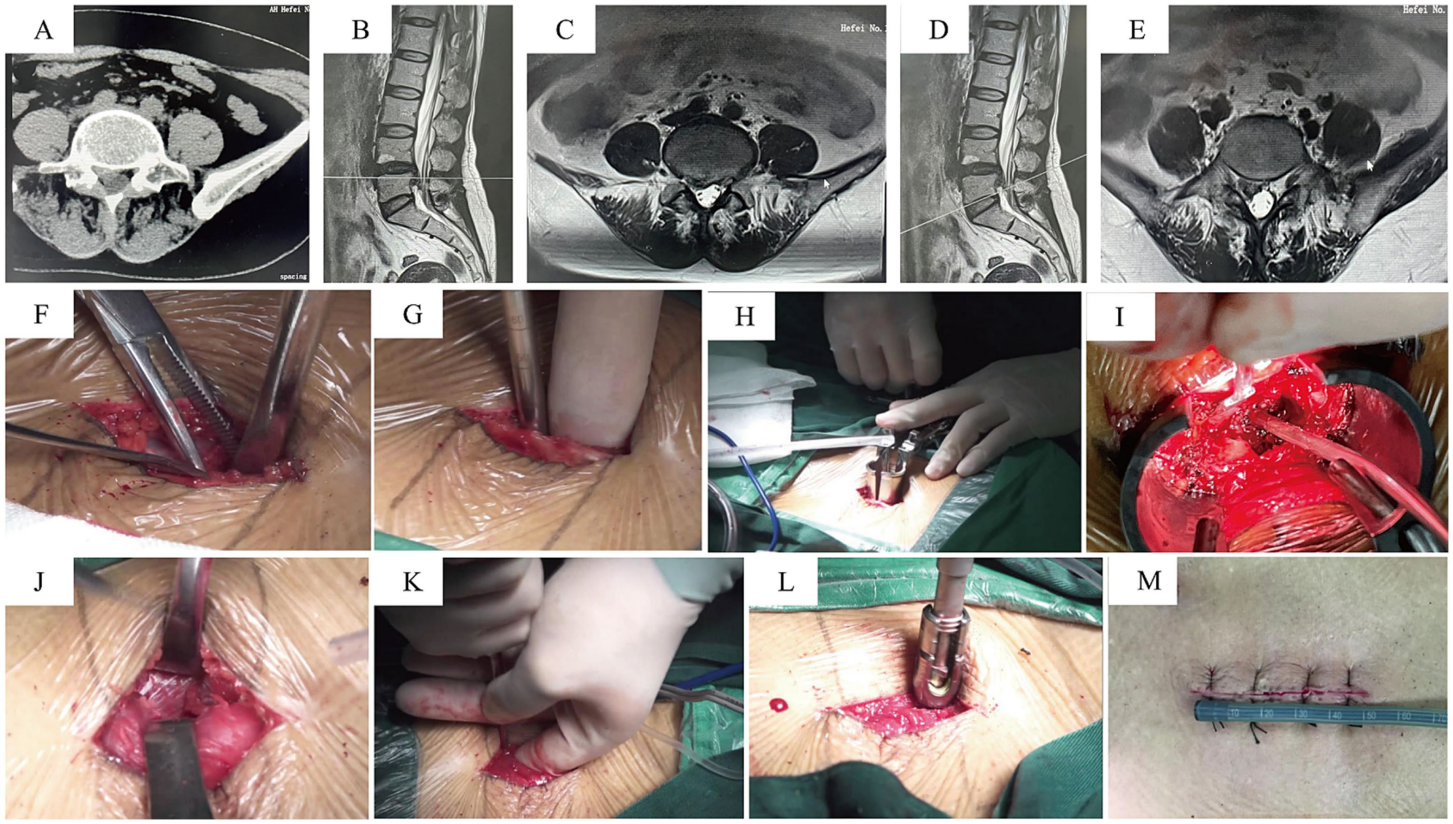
Representative Case 2. A 58-year-old female with low back pain and right lower limb numbness/pain for 2 years, aggravated for 2 months. (A–E) Preoperative CT and MRI: right-sided L4/5 lumbar disc herniation, migrated inferiorly, compressing the nerve root and causing foraminal stenosis. (F–H) A midline incision was used; the tubular retractor was inserted on the decompression side via an intermuscular plane. (I) View after completion of decompression. (J–L) Pedicle screws were placed on the contralateral side through the same midline incision via separate deep fascial incisions and an intermuscular approach. (M) Postoperative photo of the surgical wound.
